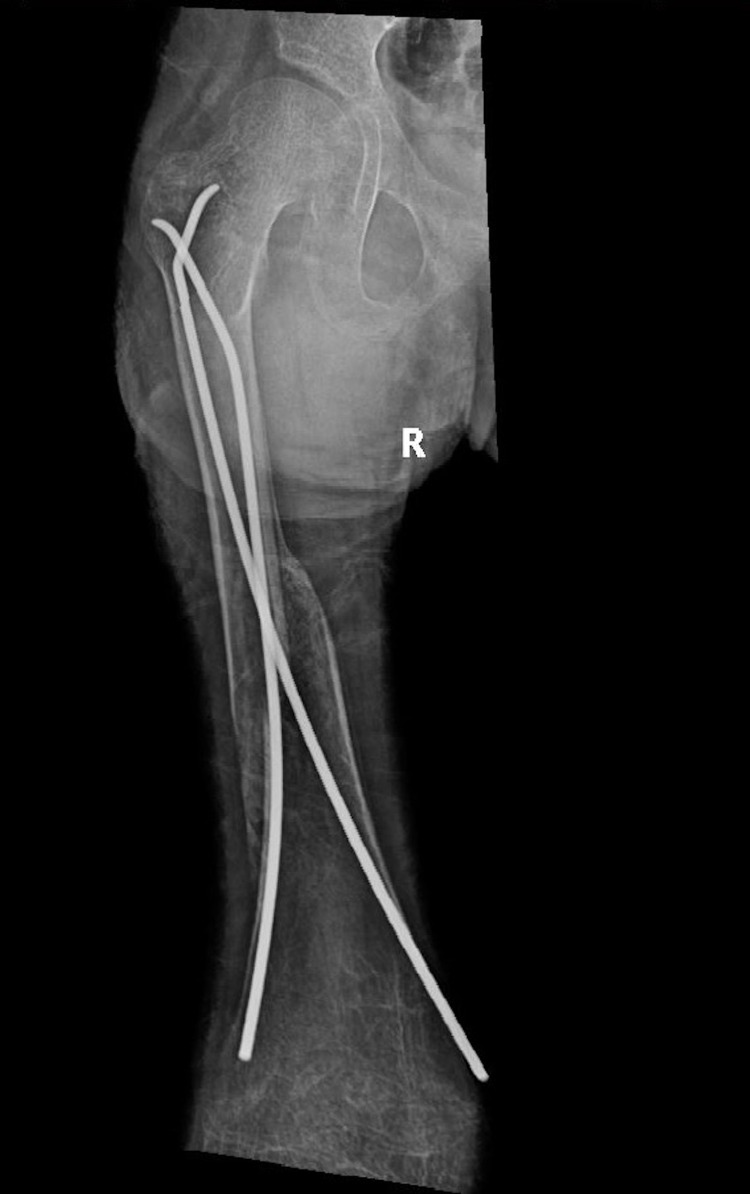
Anteroposterior view of an X-ray image of the femur eight months postoperatively with delayed healing of the femur. The Steinnman pin in the reduction with the use of the Nancy nail can be seen. There is a translation in the distal mechanical access of the femur with a 2 cm shortening because of the closed technique. Complete bone healing was established 18 weeks after the operation.
